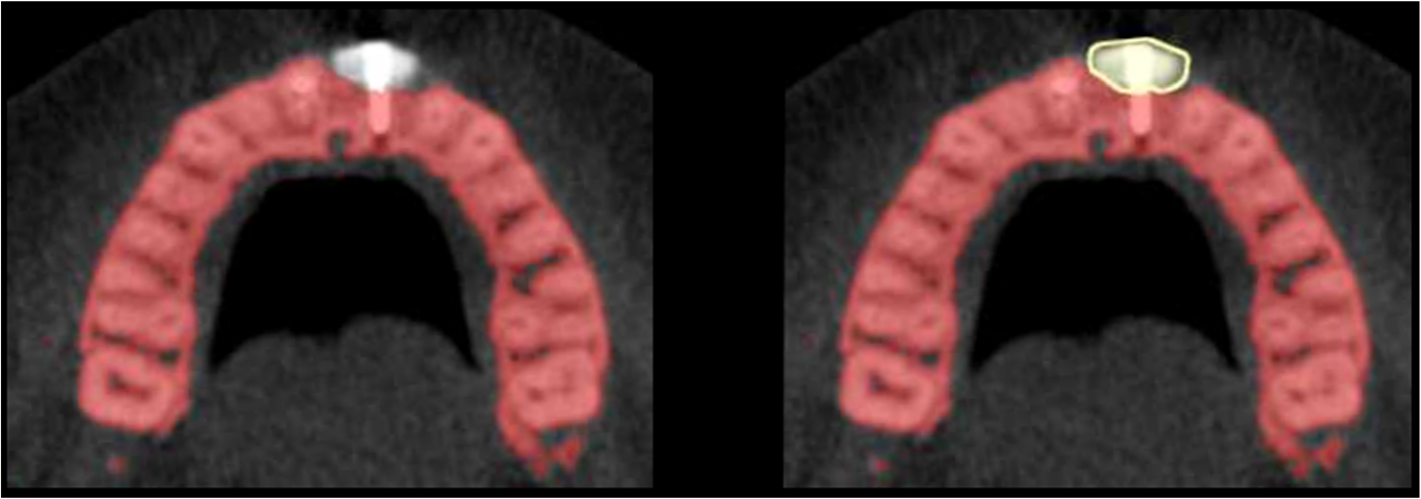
CBCT scan (axial view) of lower jaw at time point T1 postoperatively after onlay graft
